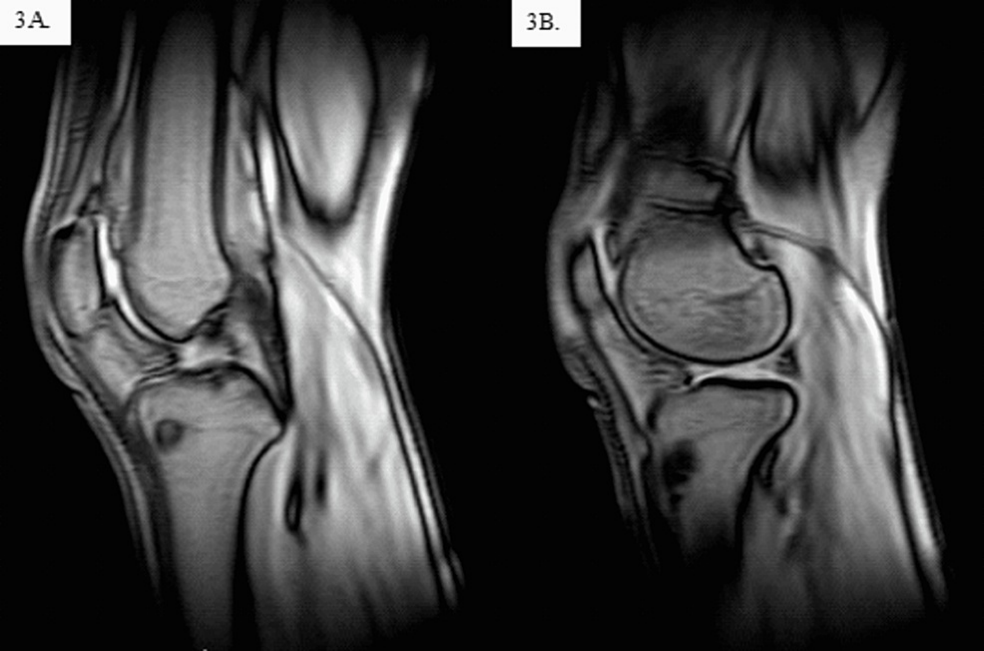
Rosai-Dorfman disease involving the proximal left tibia Magnetic resonance imaging (MRI) revealed two well-circumscribed intramedullary lesions within the left proximal tibia without cortical disruption
